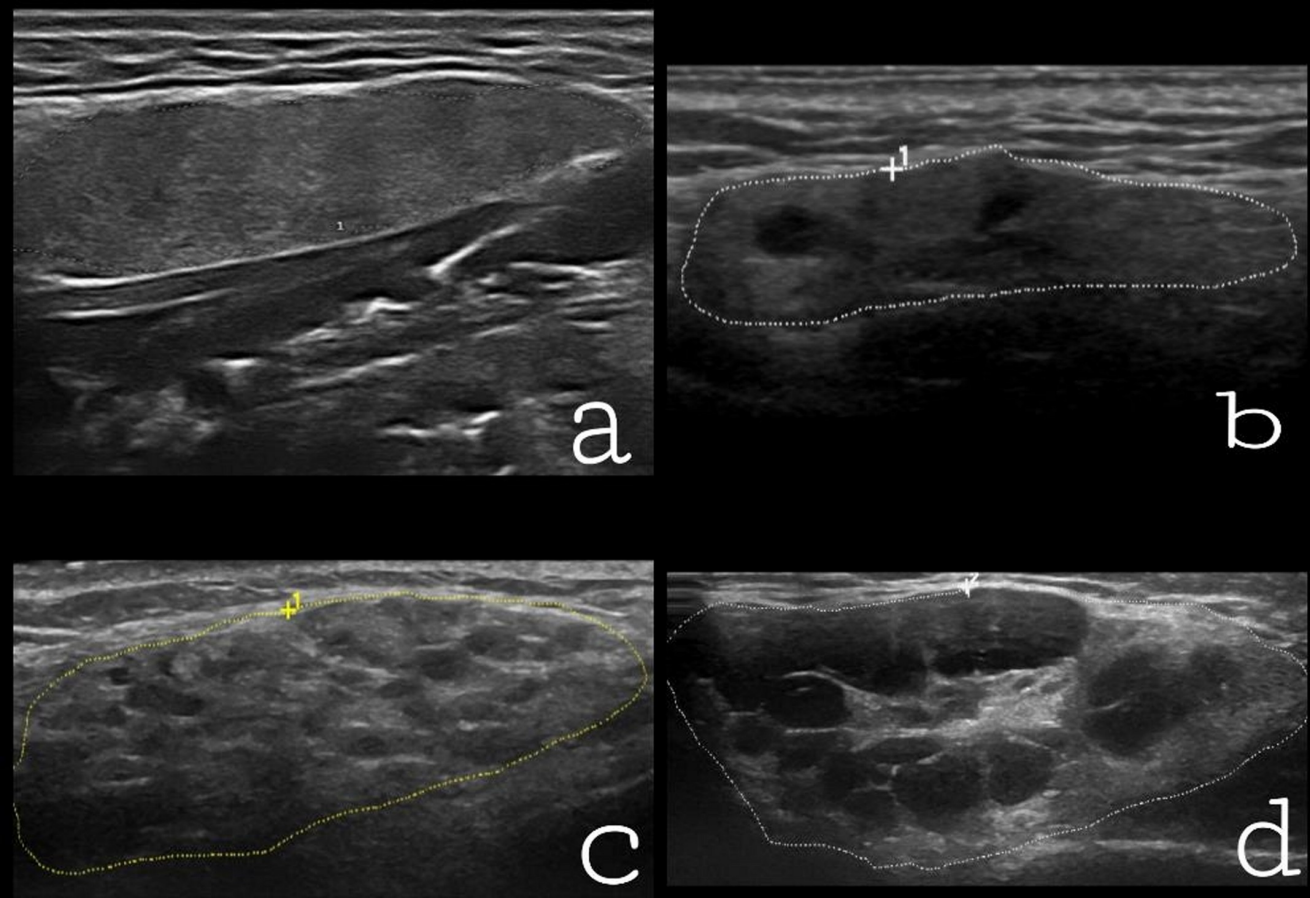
Parenchymal inhomogeneity of the salivary glands demonstrated by ultrasonography. a) Normal salivary gland (grade 0); b) a mild level of salivary inhomogeneity with isolated hypoechoic areas (grade 1); c) evident level of salivary inhomogeneity (grade 2); and d) gross level of salivary inhomogeneity (grade 3).
